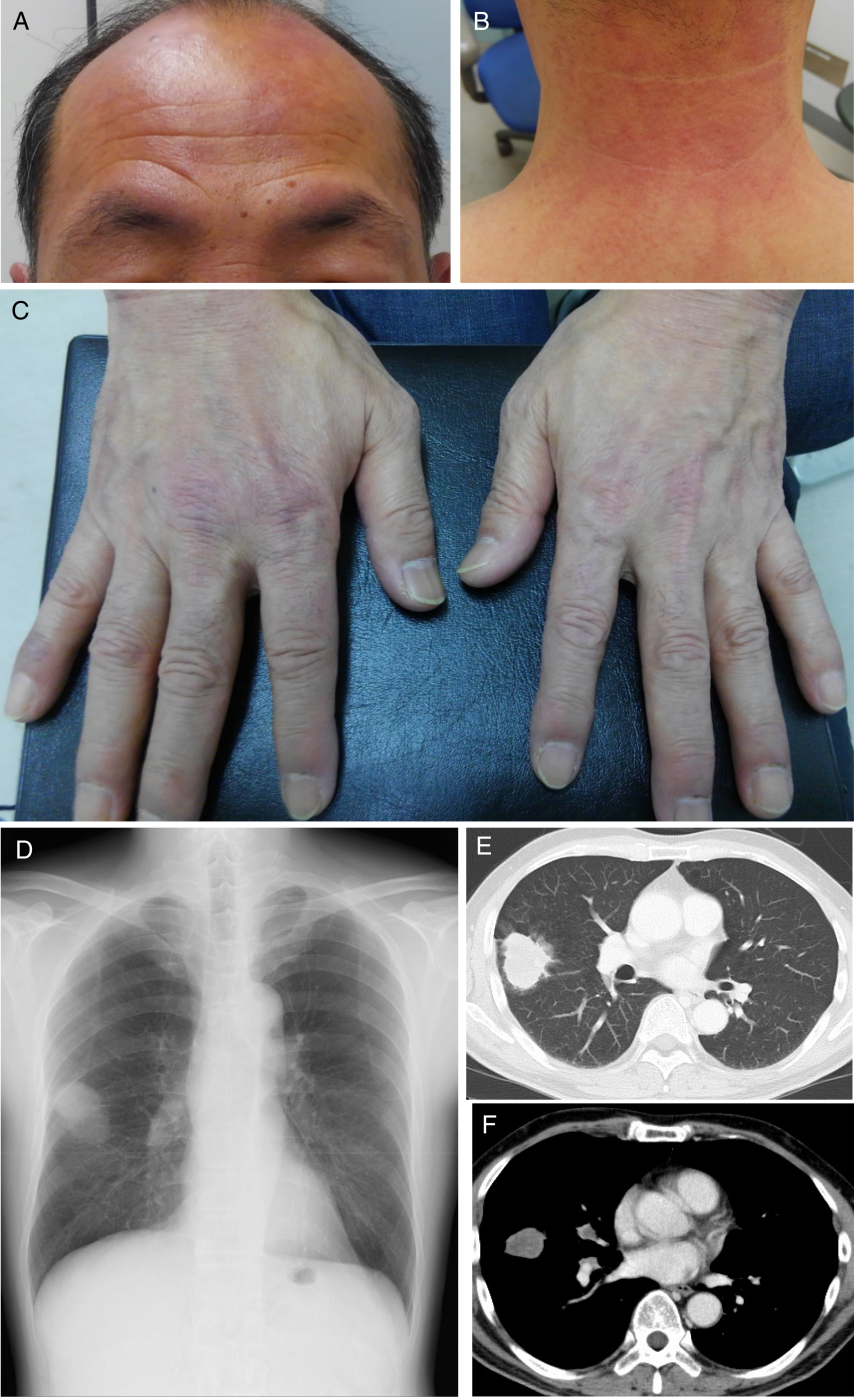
Macular rash on his forehead and swelling of upper eyelids (A), suggestive of a heliotrope rash. Shawl sign was recognized characterized by a widespread, flat, reddened area on his upper back, shoulders, and posterior neck (B). A flat, red rash on the back of the fingers and hands, indicating a Gottron’s sign (C). Chest X‐ray demonstrated the mass as large as 4 cm in diameter in the right middle lung fields (D), which was confirmed by thoracic computed tomography depicted as an inhomogeneously enhanced solitary mass (4 cm in size) in the right upper lobe (E), with ipsilateral hilar lymphadenopathy (F).
